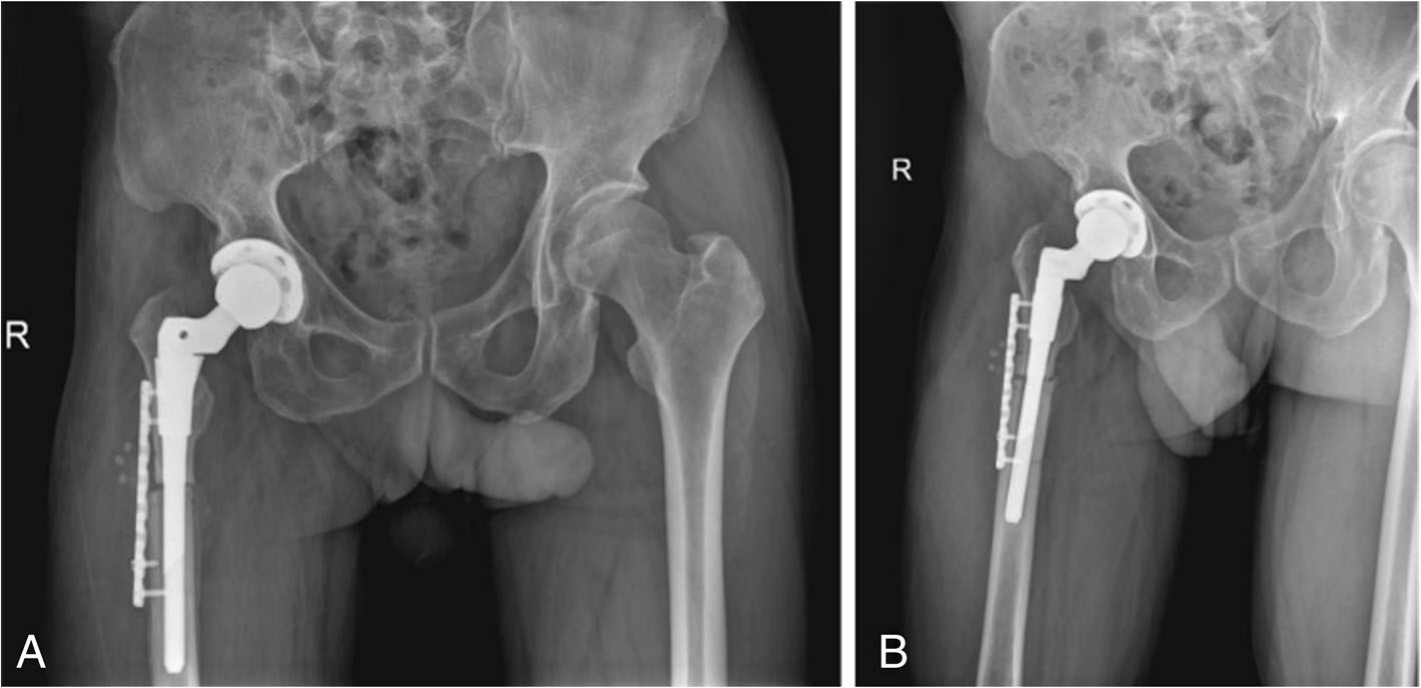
Radiographs of the right hip at 1 month after internal fixation was applied. (a) anteroposterior view of the right hip, (b) lateral view of the right hip
